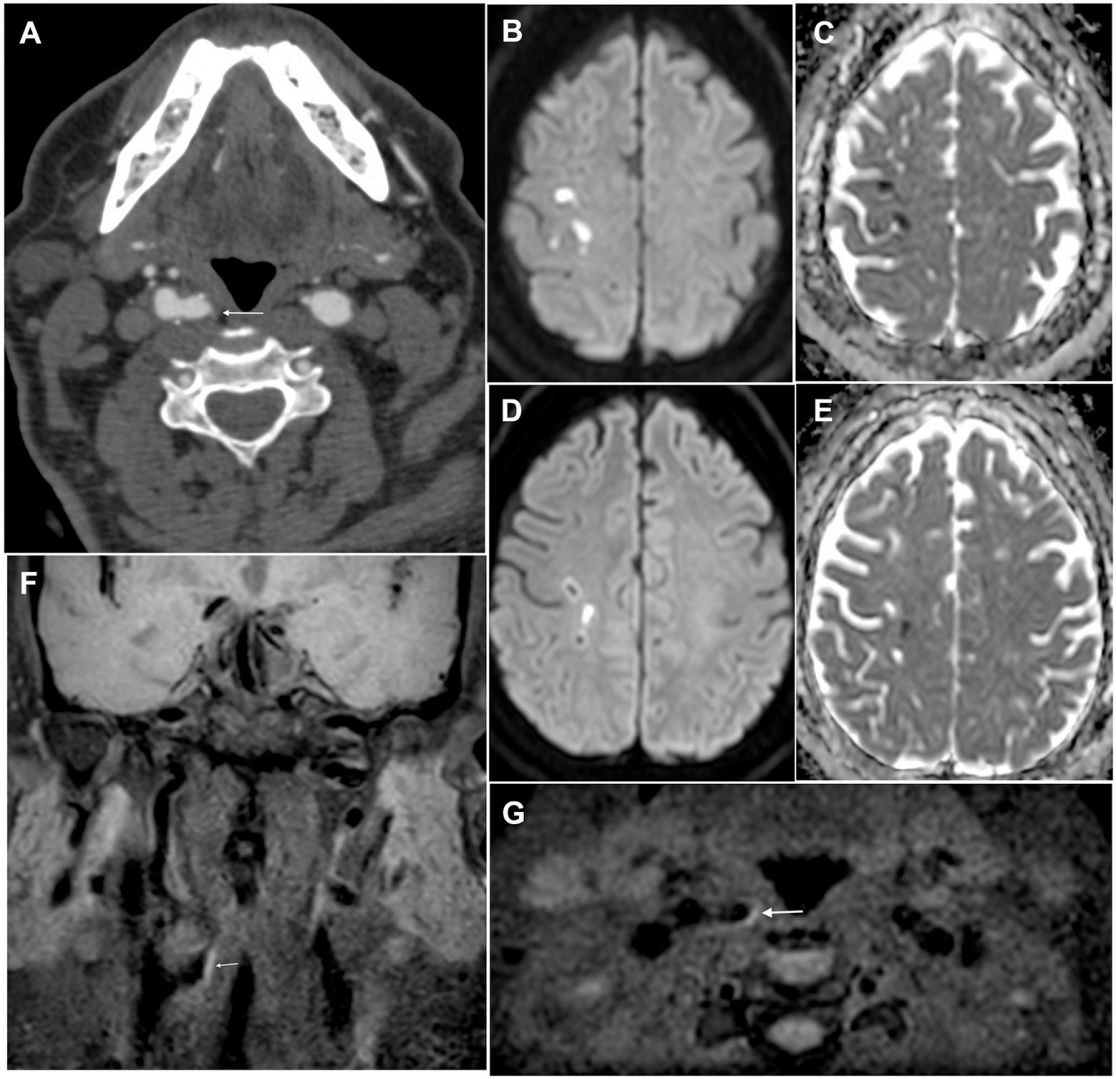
Brain and plaque imaging findings in a 64-year-old man with ESUS. (A) Axial angio-CT scan slice showing a hypodense non-stenotic carotid plaque in the right internal carotid artery (white arrow). (B–E) Axial diffusion-weighted imaging slices (with corresponding ADC maps) showing multiple embolic strokes in the right pre-and post-central area. (F,G) Coronal and axial T1-weighted black blood sequence showing hyperintensity of the non-stenotic plaque in the right internal carotid artery (white arrow), thus confirming the presence of intraplaque hemorrhage.
